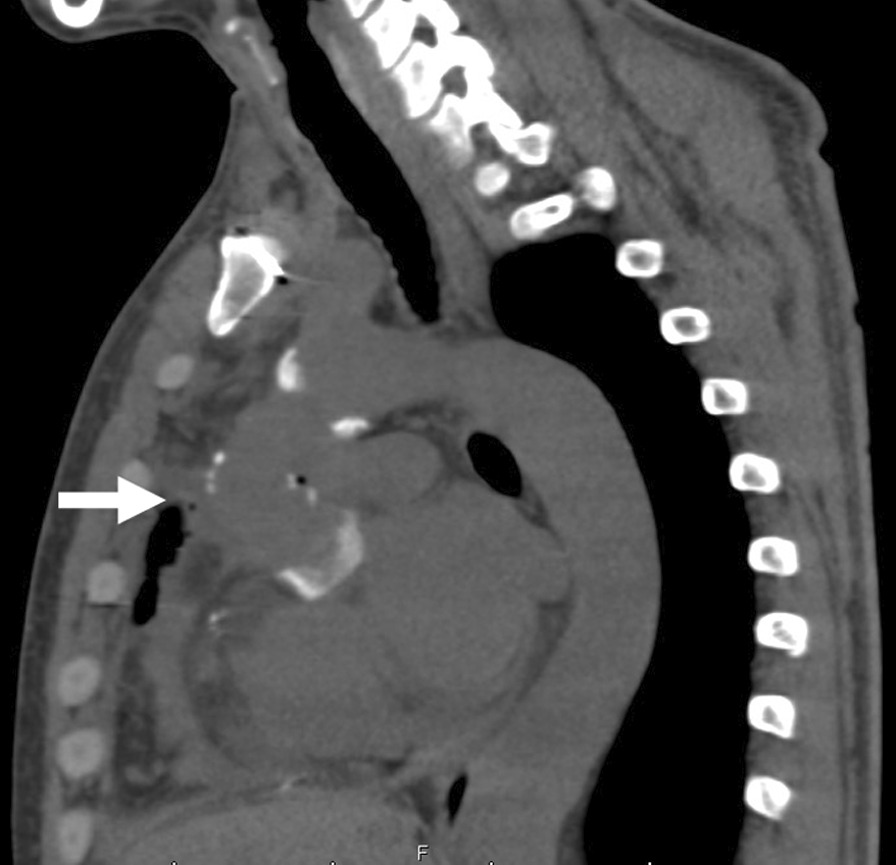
Chest CT scan 5 days after redo surgery showed no obstruction in the ascending aorta (arrow)
